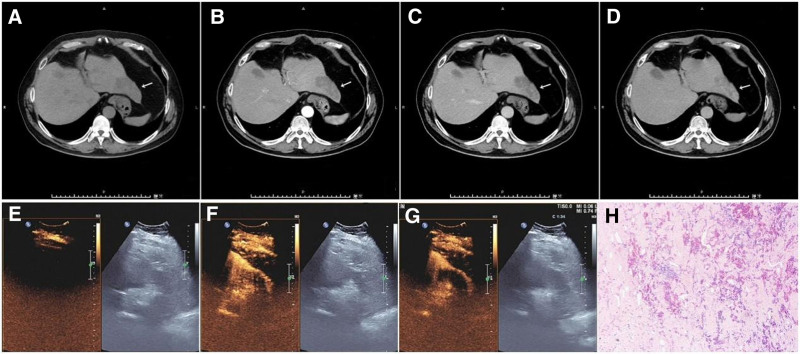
CT and CEUS manifestations of AH. (A) Plain CT scan. (B) Arterial phase. (C) Portal venous phase. (D) Delayed phase. (E) Arterial phase (CEUS). (F) Venous phase (CEUS). (G) Delayed phase (CEUS). (H) Pathological image. CEUS = contrast-enhanced ultrasound, CT = computed tomography.
